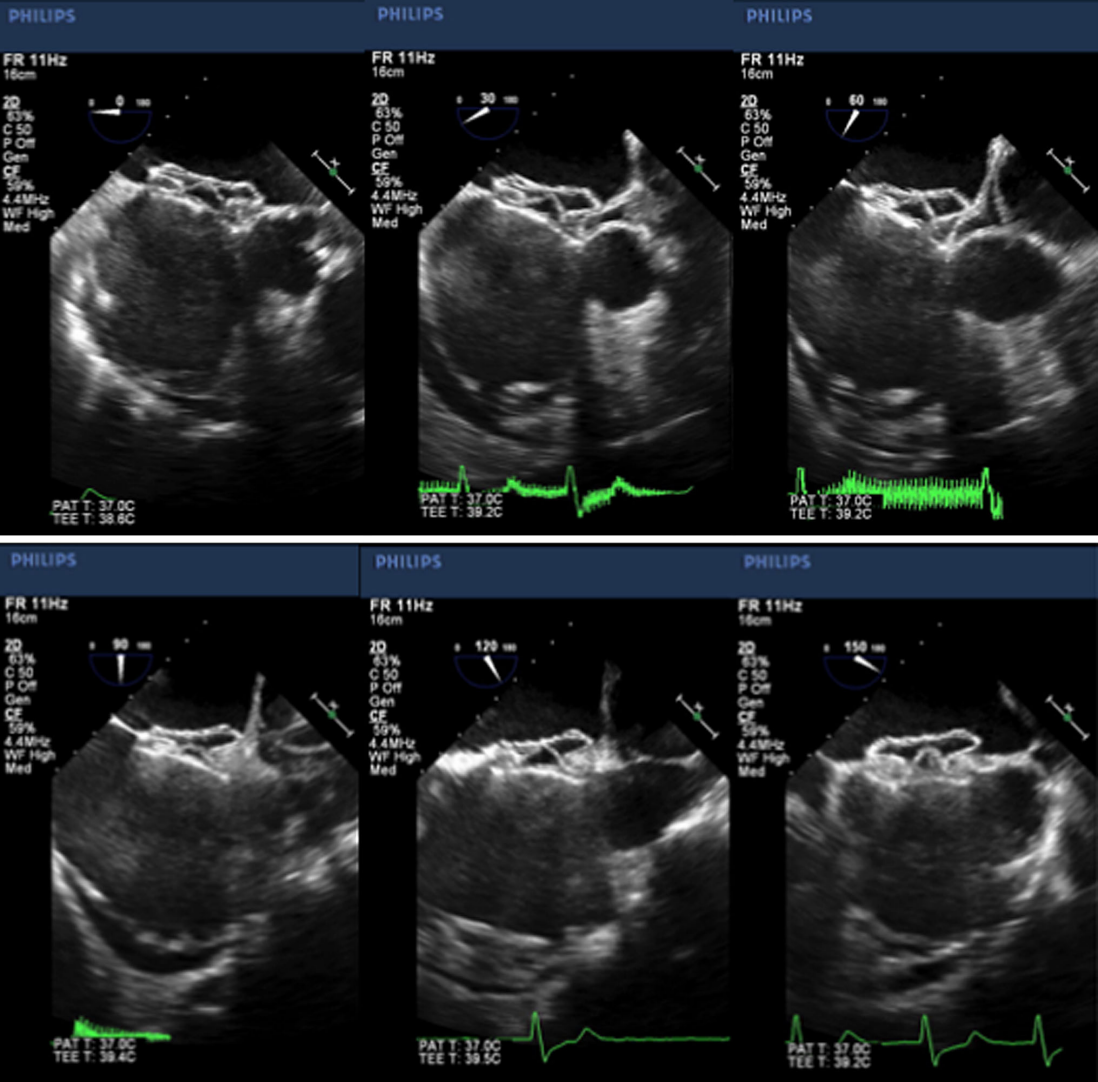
Transesophageal echocardiographic (TEE) image after deploying the Figulla Flex II ASD occluder. The device was stable, and TEE at 30° and 60° views showed good device conformability with the malaligned septum and minimal left disc tenting of the atrial free wall into the transverse sinus
